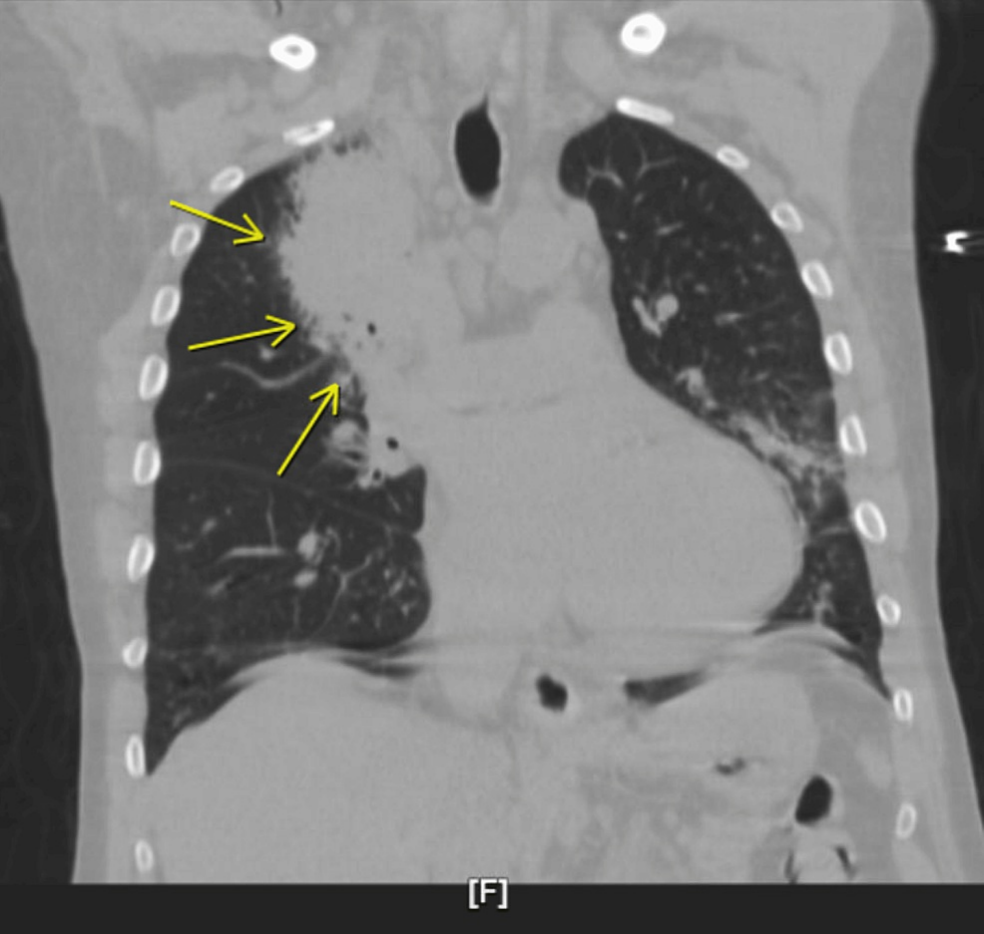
CT chest showing right lung mass.
